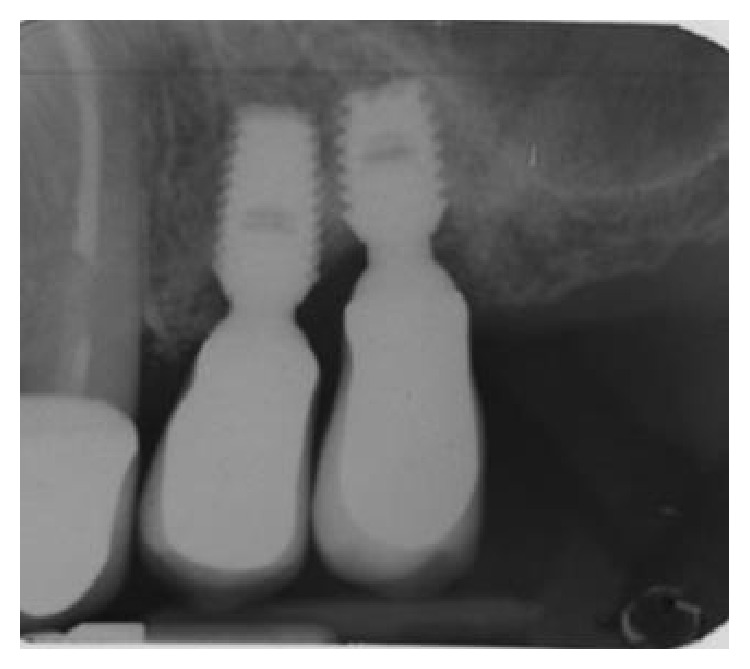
Two-year postoperative radiographs depicting radiopacity at the location of the defect, showing a near complete regeneration of the missing bone.
